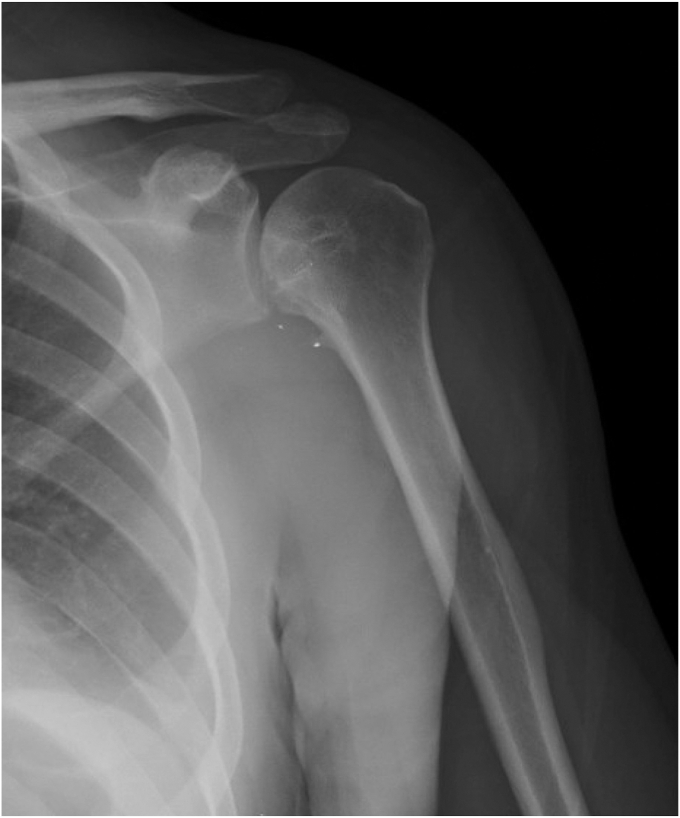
Stable, healed OCA on radiographic imaging at 20 months postoperative visit. *OCA*, osteochondral allograft transplantation.
